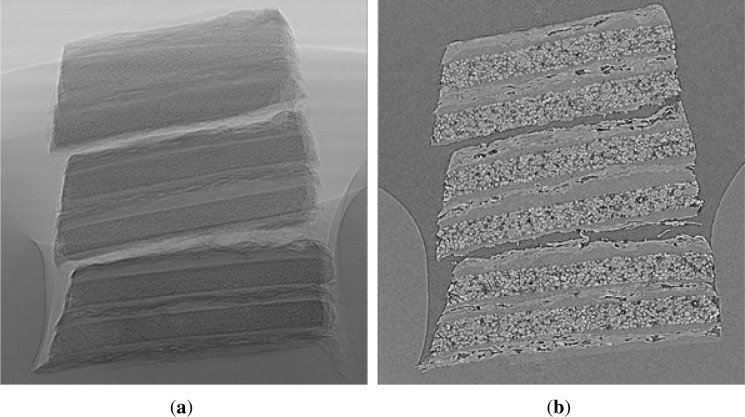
2D images of a sample stack. (a) Radiographic projection image; (b) reconstructed image.
